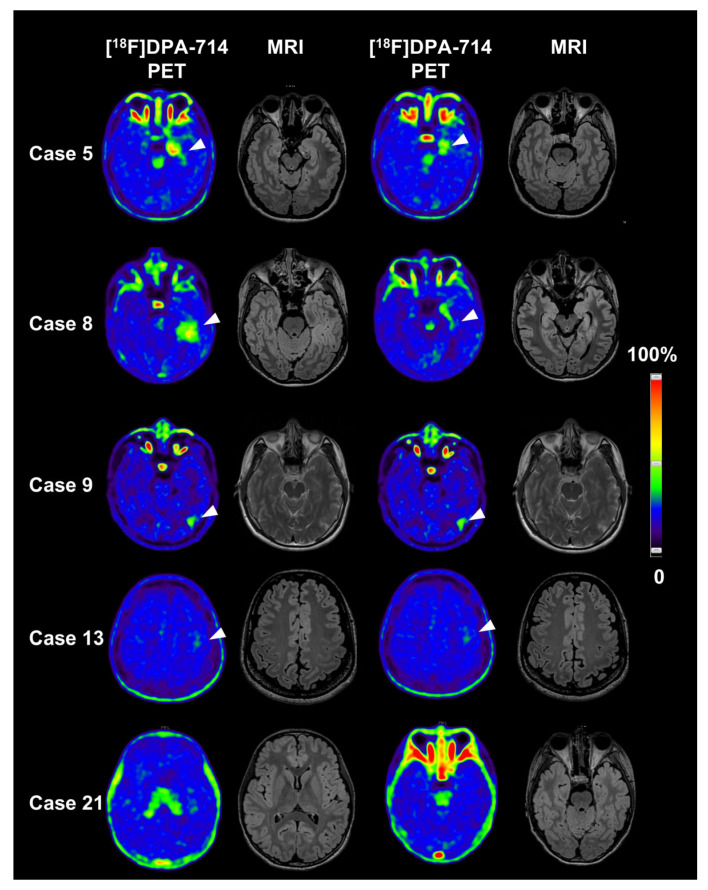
Representative TSPO-PET/MRI pattern in patients with TLE. Case 5 and Case 8 presented focal high [^18^F] DPA-714 uptake in left temporal lobe (white arrowhead) while Case 9 exhibited focal high uptake in left occipital lobe (white arrowhead). Case 13 showed a regional higher signal in left frontal lobe. Case 21 displayed very low or visually undetectable uptake. TSPO, translocator protein 18 kDa; PET, positron emission tomography; MRI, magnetic resonance imaging; TLE, temporal lobe epilepsy.
